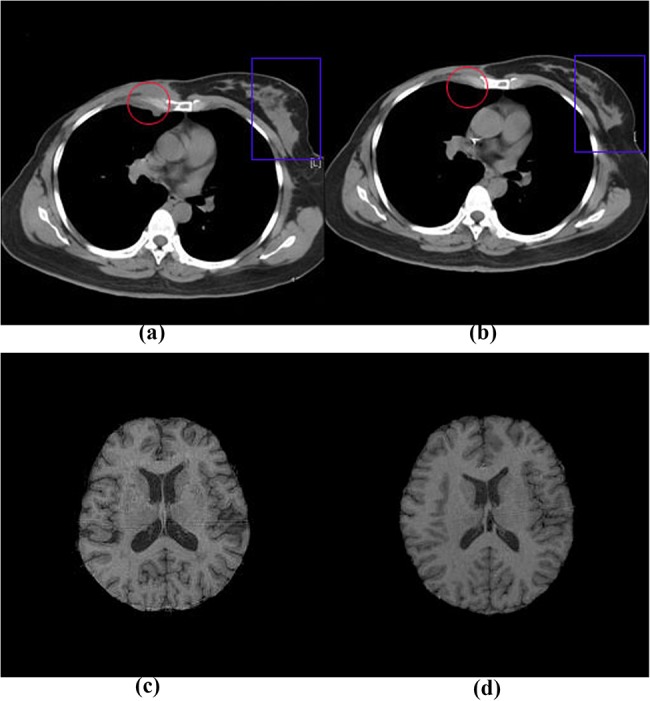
Medical images examples for method evaluation. (a) reference image of chest CT image pairs,(b) floating image of chest CT image pairs, (c) reference image of brain MR image pairs, (d) floating image of brain MR image pairs.
